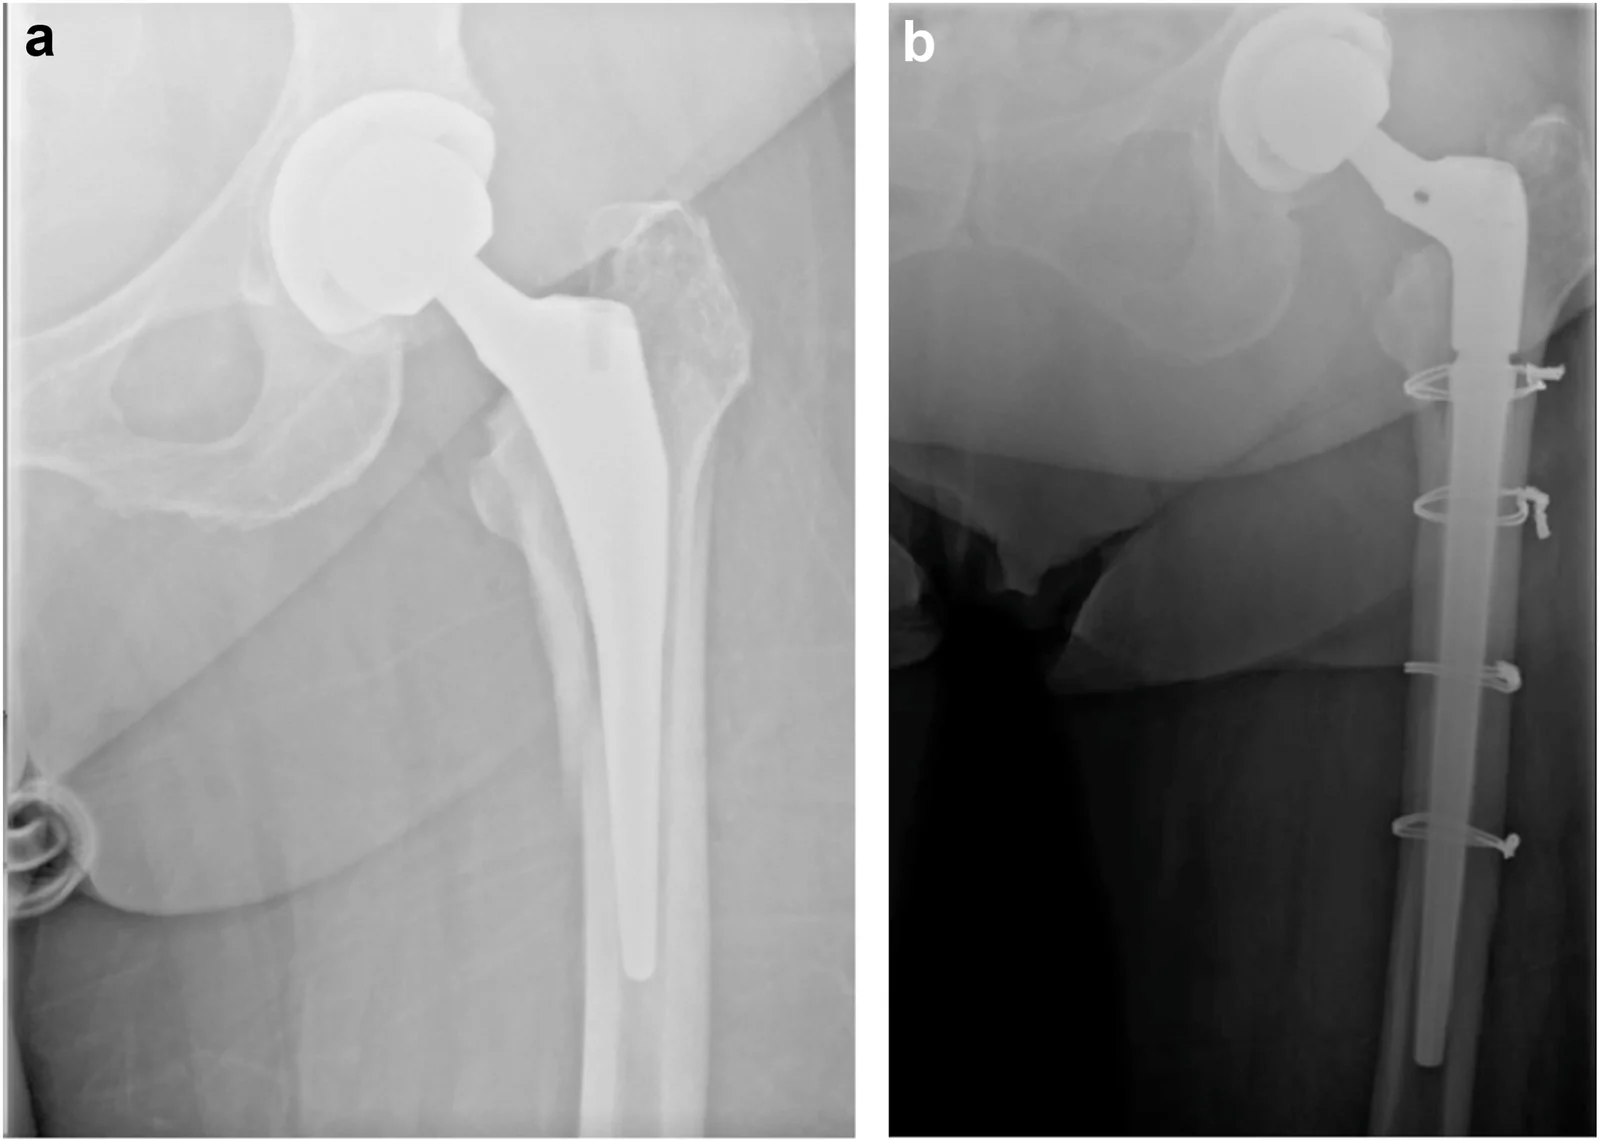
Anteroposterior pelvis radiographs demonstrating (a) a Vancouver B periprosthetic fracture and (b) treatment with a splined tapered stem secured with multiple cerclage wires placed using the described technique.
